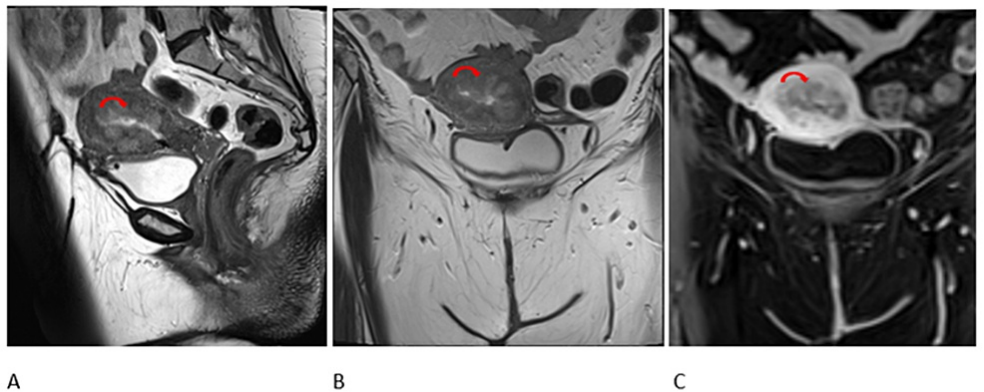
Sagittal T2 (A), coronal T2 (B) and post-contrast T1 (C) MRI images through the pelvis show irregular lobular mass lesions around the endometrial cavity and extending into the myometrium (red arrows).
